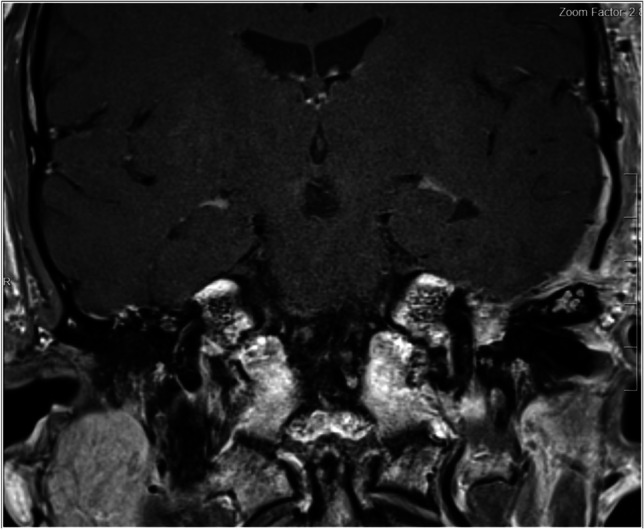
Postoperative gadolinium contrast-enhanced T1-weighted MRI in the coronal plane
